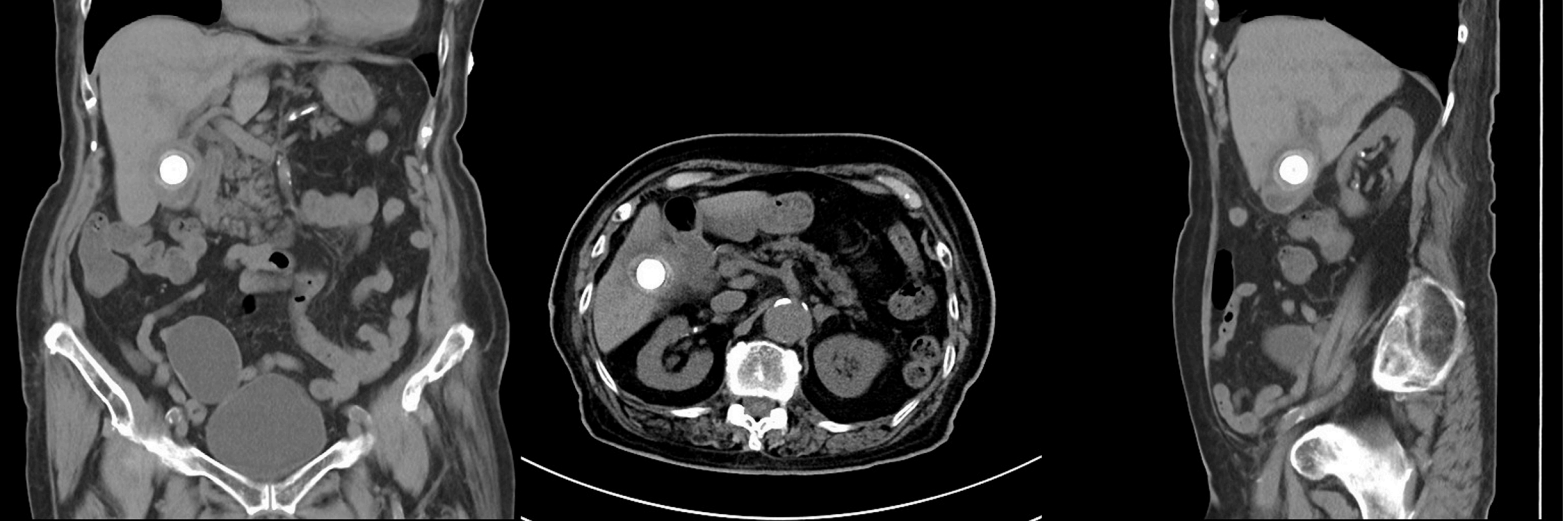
Abdominal computed tomography scans 1 year prior showing the gallstone in the gallbladder adjacent to the duodenum.
